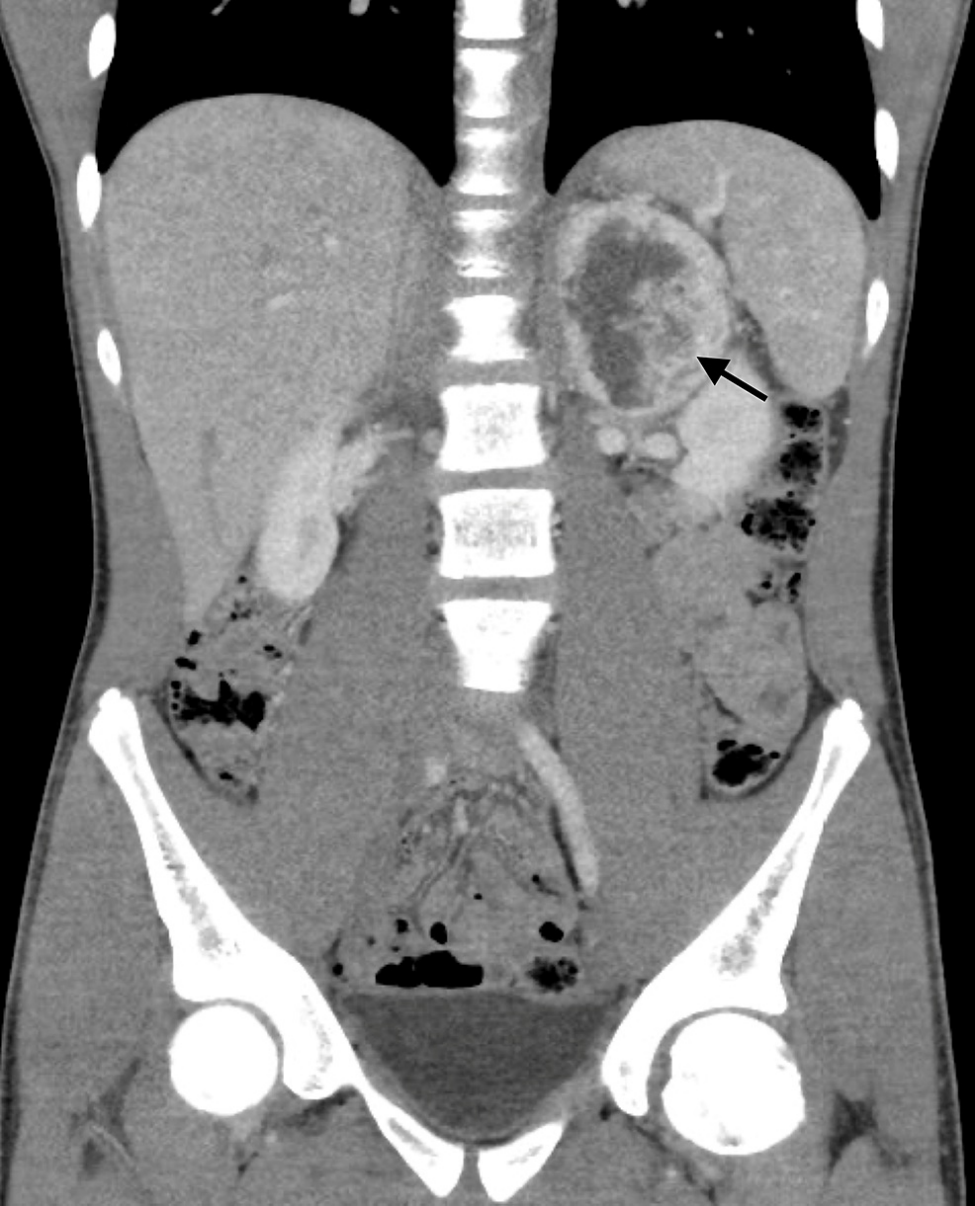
Coronal MPR CT image with contrast demonstrating heterogeneously enhancing left adrenal mass (black arrow). MPR: multiplanar reformation; CT: computed tomography
